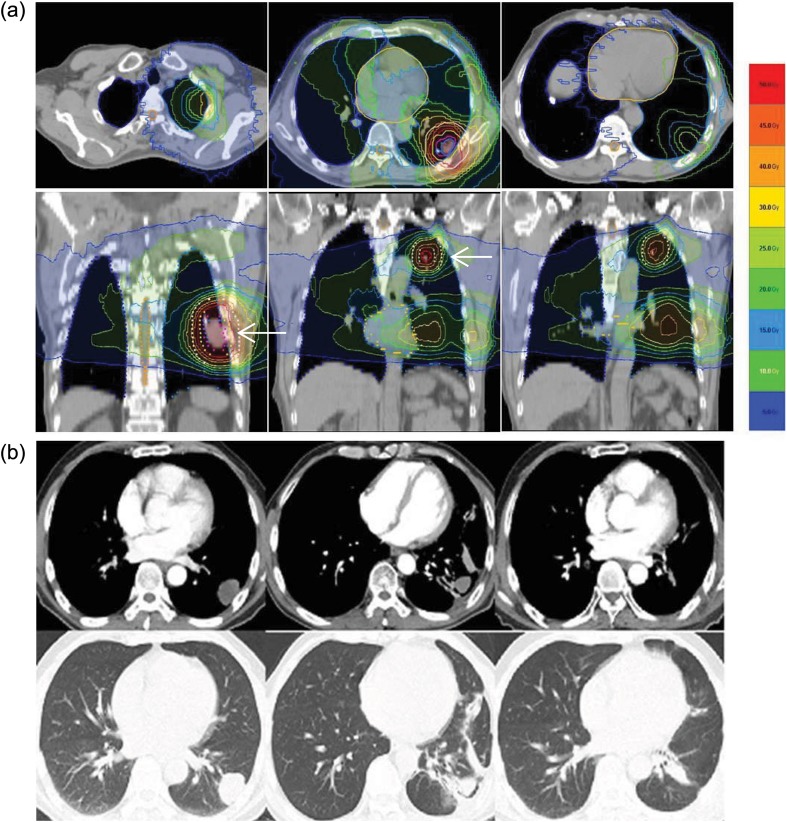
Case presentation: a 60-year-old man with multiple lung metastases (×4) from hard palate cancer. The primary site was surgically removed five years ago. After detection of multiple lung metastases, the patient was treated with systemic chemotherapy and the response was stable. Treatment with hypofractionated radiotherapy using helical tomotherapy was decided upon. The prescriptive dose was 50 Gy to the ITV (internal target volume) and 40 Gy to the PTV (planning target volume) in 10 fractions over 2 weeks. (a) The treatment plan with isodose lines superimposed on the planning computed tomography. The following isodose lines are shown: 50 Gy, 45 Gy, 40 Gy, 30 Gy, 25 Gy, 20 Gy, 15 Gy, 10 Gy and 5 Gy. The PTV of two metastatic nodules in the left lung was surrounded by the 40 Gy line white arrows. However, the 10% isodose line (5 Gy) surrounded almost the entire lung area. The mean lung dose and V5 for this patient were 14.98 Gy and 88%, respectively. (b) The computed tomography before radiotherapy showed a metastatic nodule in the left lower lung, and the total number of lung metastases was four (not shown) (two images on the left). The computed tomography three months after radiotherapy showed radiation pneumonitis. The patient complained of a dry cough but did not require medication, so we categorized this as Grade 2 RP. The morphologic changes due to RP, as seen in the CT scan, were limited to V30, a small volume of the lung receiving a relatively high dose, therefore, symptoms such as the dry cough might have been caused by the large distribution of the low dose, for example V5 (central two images). One year after completion of radiotherapy, the computed tomography showed minimal radiation fibrosis, and the patient did not complain of respiratory-related symptoms (two images on the right).
